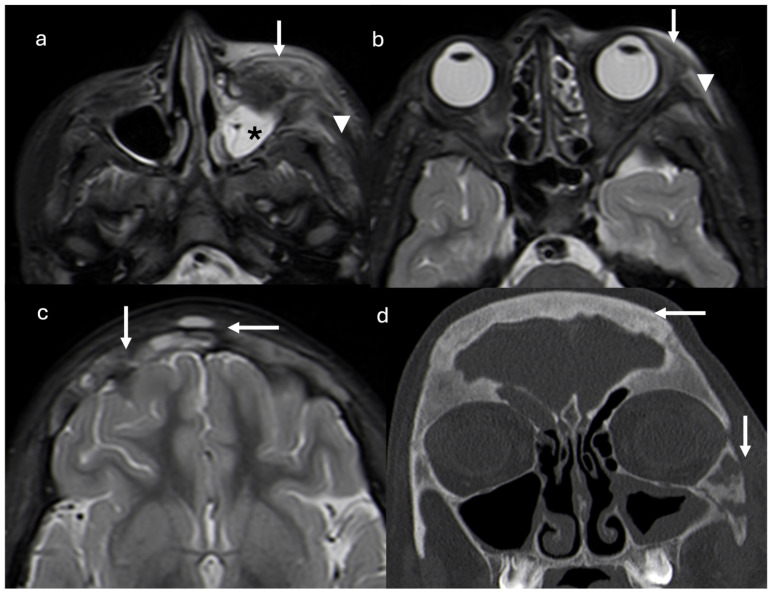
Axial short tau inversion recovery (STIR) magnetic resonance images show diffuse mucosal thickening of left maxillary sinus (asterisk), periorbital adipose tissue inflammation and pre-septal cellulitis (arrows), and temporal and masseter muscle edema (arrowheads) (a,b). Thickening and diffuse signal alterations of frontal bone with epicranic soft tissue involvement (arrows) are demonstrated (c). Coronal reconstruction CT scan (d) confirmed thickening on frontal and zygomatic bones with lytic areas. Right frontal and left maxillary sinus inflammation is present (arrows).
